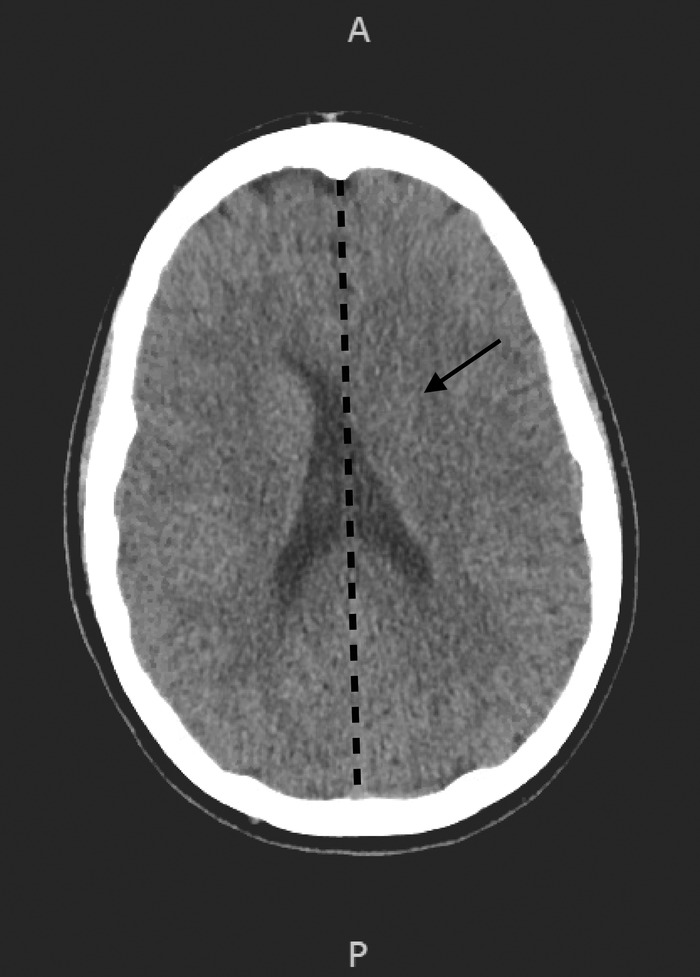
Computed tomography of the head without contrast upon arrival shows left basal ganglia hypodensity with mass effect on the left lateral ventricle (arrow) and 10.5-mm rightward shift of midline structures (midline depicted by dotted line).
